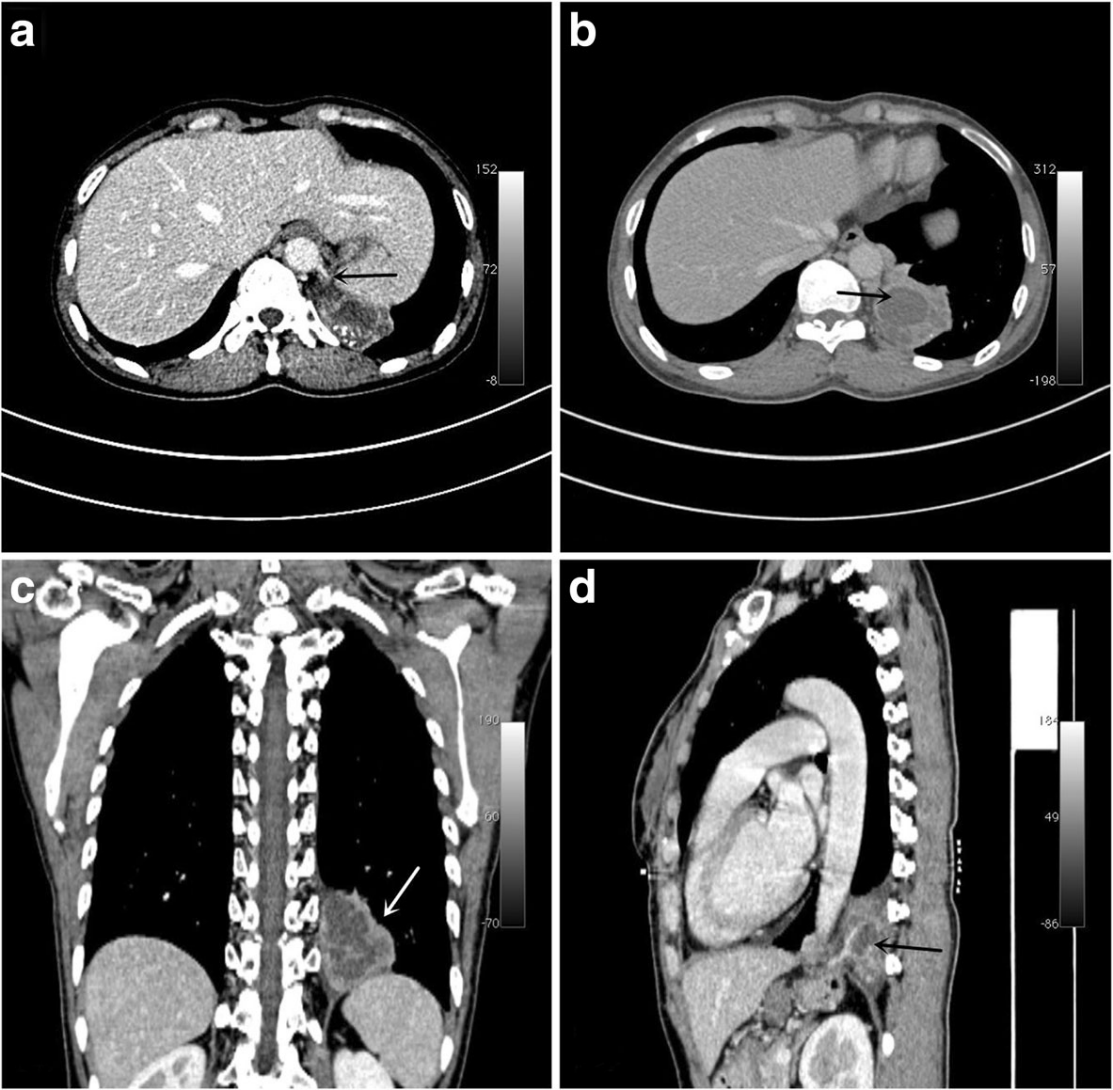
a Enhanced CT scan: abnormal blood vessels from the abdominal aorta; b mass and abnormal blood vessels in the mediastinum; c coronal plane; and d sagittal plane
